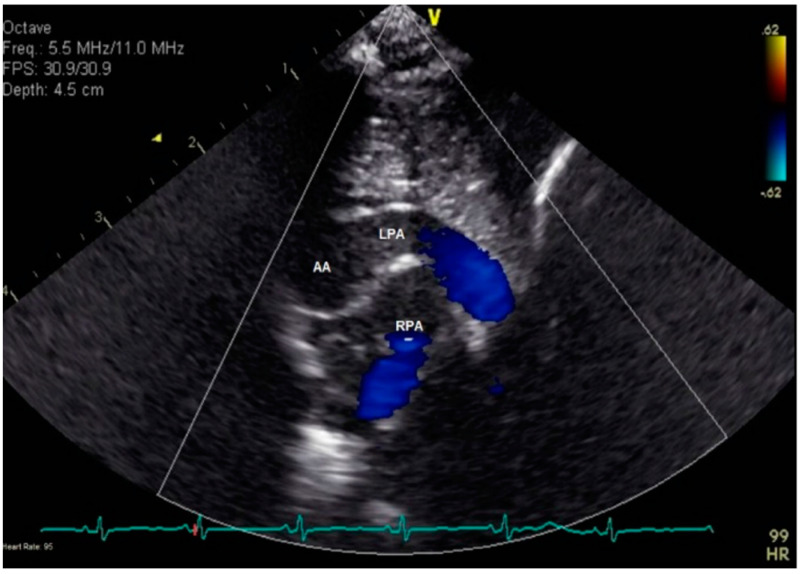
Echocardiography in the high-right parasternal short-axis view in a more superior plane than Figure 2. Color interrogation at this level demonstrates a vessel arising from the anterior aspect of the ascending aorta and then coursing leftward, passing over the pulmonary trunk and the right pulmonary artery.
